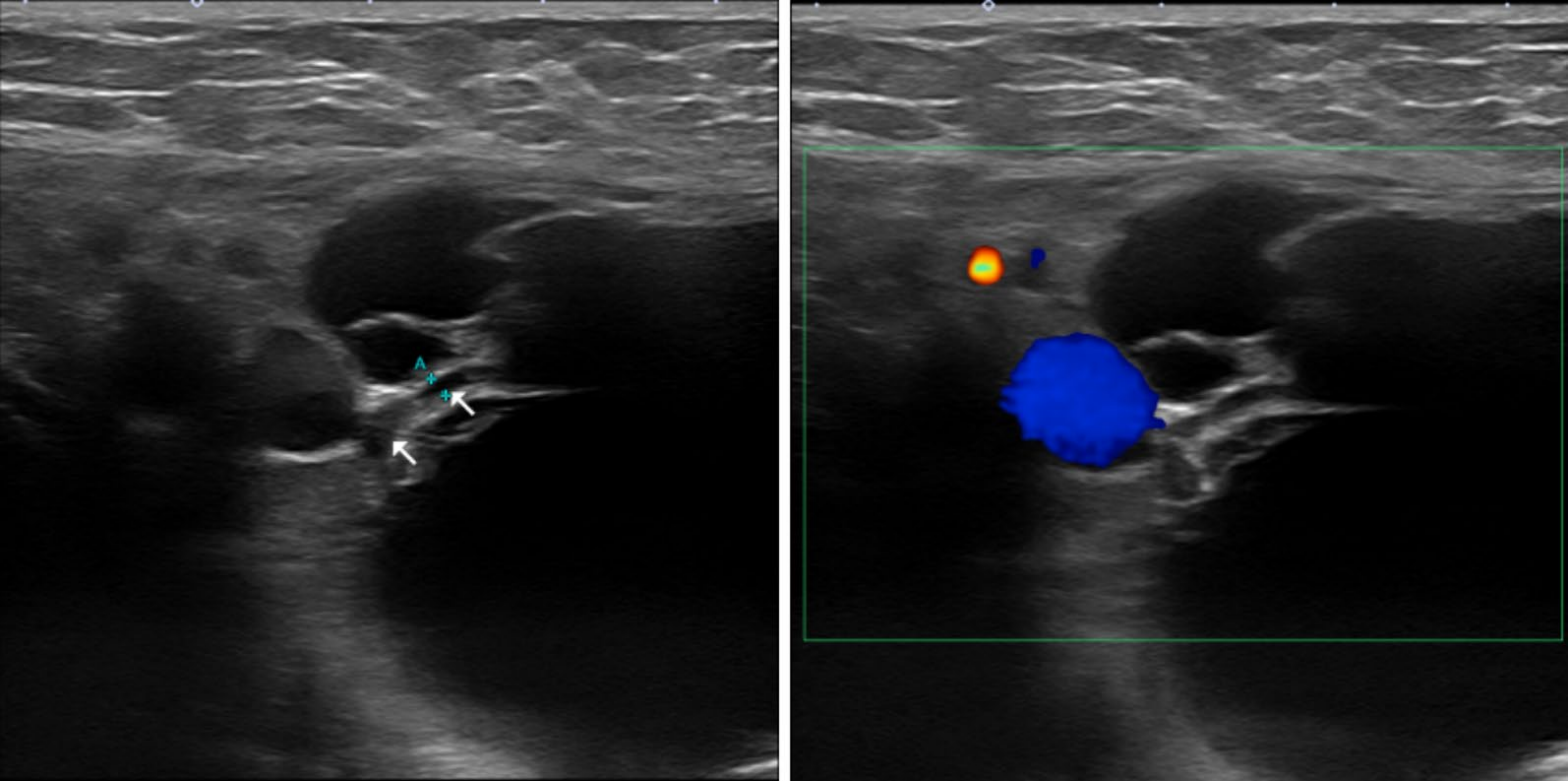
Breast ultrasonography findings. Breast ultrasonography confirmed a multilobulated cystic mass in the left axial region and branches (arrow) from the mass connected to the axillary vein
